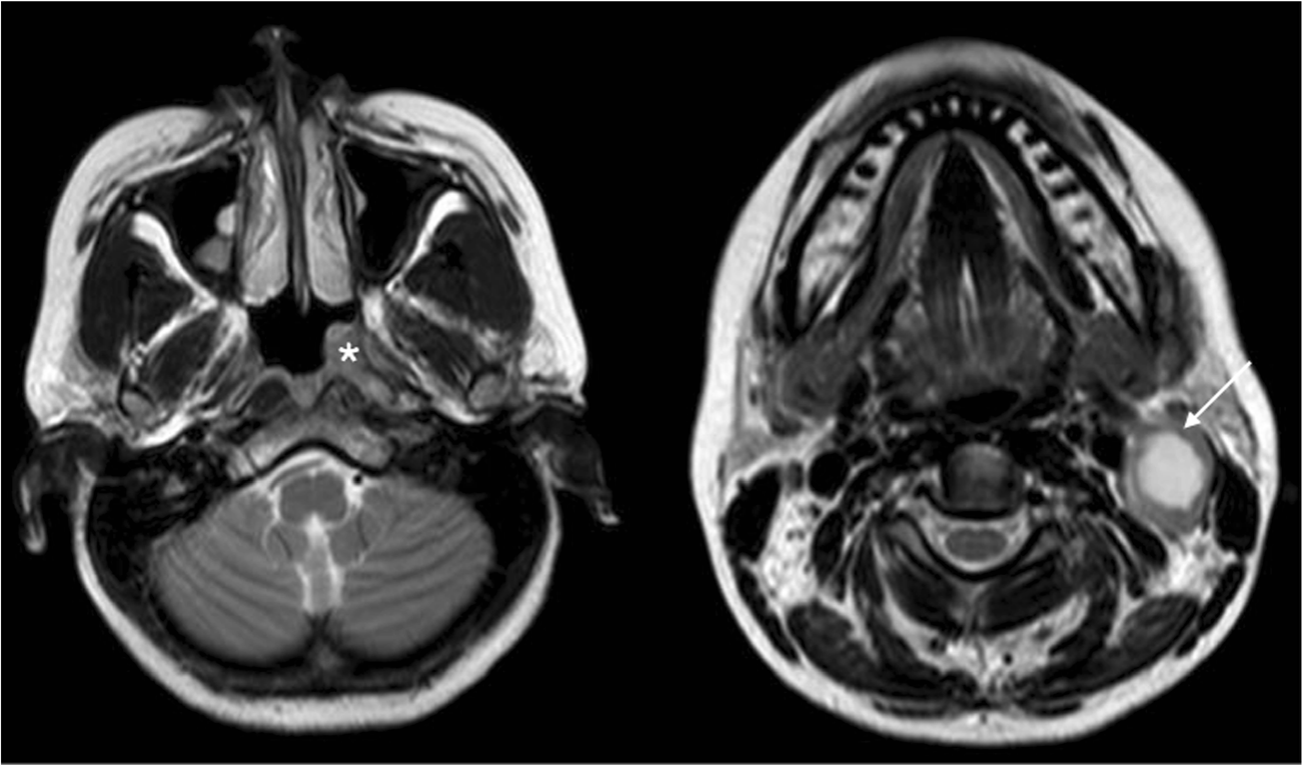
Forty-year-old man presenting with a persistent level II neck lump. Axial T2-weighted imaging demonstrates a complex cystic lesion posterior to the left angle of the mandible (*white arrow*). Asymmetrical soft tissue was also appreciated in the left fossa of Rosenmüller (*asterisk*). A second branchial cleft cyst was considered in the differential, but the diagnosis was finally confirmed to be nasopharyngeal carcinoma with cystic metastases
